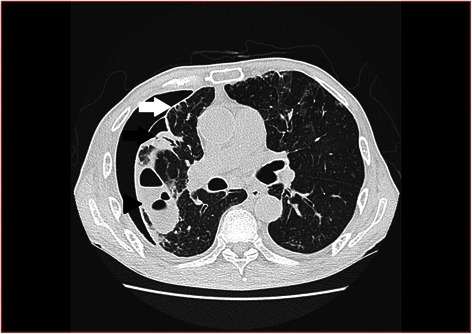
Chest computed tomography shows thickened visceral pleura (white arrow) and an alveolarpleural fistula (APF) (black arrow) at the lateral aspect of the right upper lobe with capsulized interlobar effusion (arrow head)
